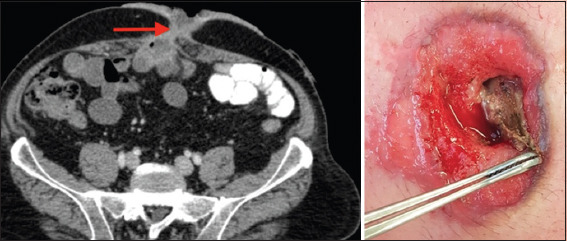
Abdominopelvic computed tomography scan (left) displaying an anterior enterocutaneous fistula (red arrow) at the site of a previous hernia repair with synthetic mesh. Photo of the fistula (right) with the exposed mesh.
